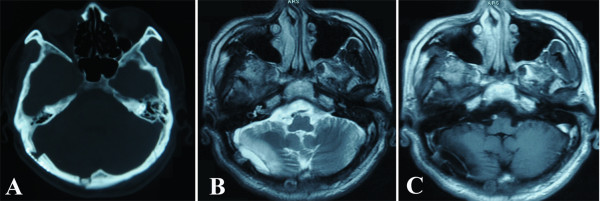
Follow-up radiological images 17 months after the operation. These images reveal that the right occipital intracranial mass has disappeared. A, unenhanced axial CT image; B, unenhanced axial T2-weighted MR image; C, enhanced axial MR image.
